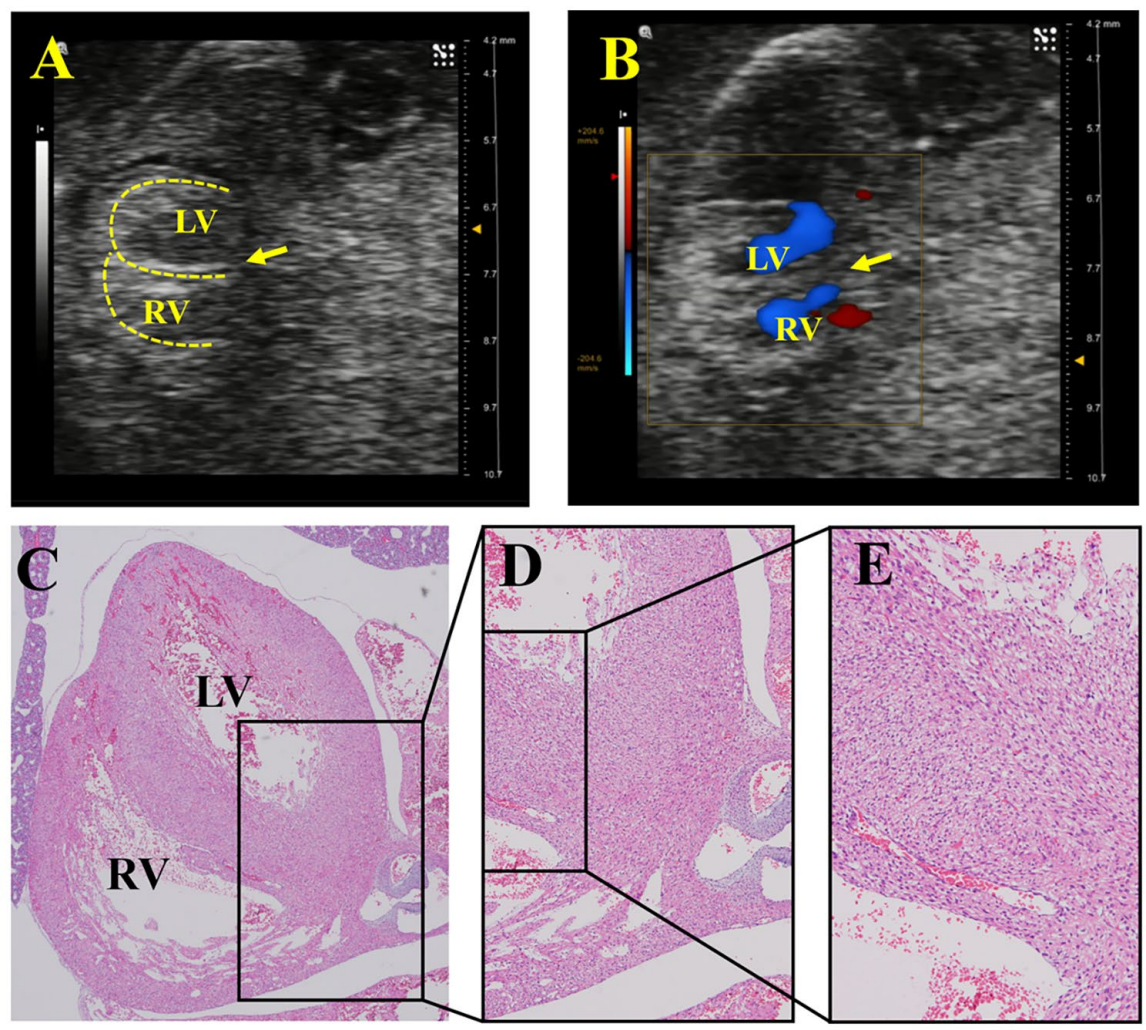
Prenatal cardiac ultrasound and pathological images of normal fetuses. A is a B-mode image, where the echoes of the ventricular wall and septum can be observed. B is a color Doppler image, and no transseptal blood flow was observed. C, D, and E are all pathological images (magnification are 40×, 100× and 200×, respectively), with an intact ventricular septum and tightly arranged cardiomyocytes of the ventricular septum. (The dotted line indicates the ventricular wall and septum, and the arrow points to the ventricular septum. LV, left ventricle; RV, right ventricle)
